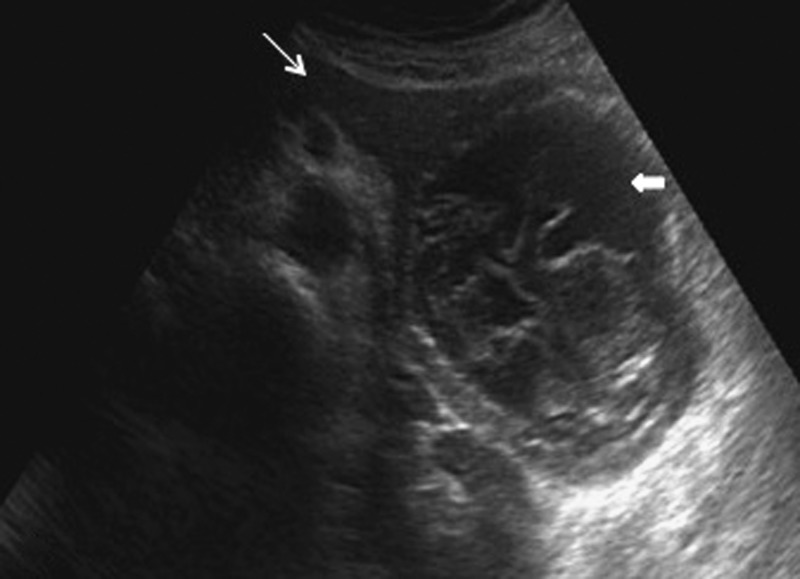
Ultrasound sonography image showing a well-defined anechoic lesion with thick double-lined hyperechoic wall and internal echogenic serpentine structures arising from the distal body and the tail of the pancreas (bold white arrow). The proximal body and the head of the pancreas appear normal (thin white arrow).
